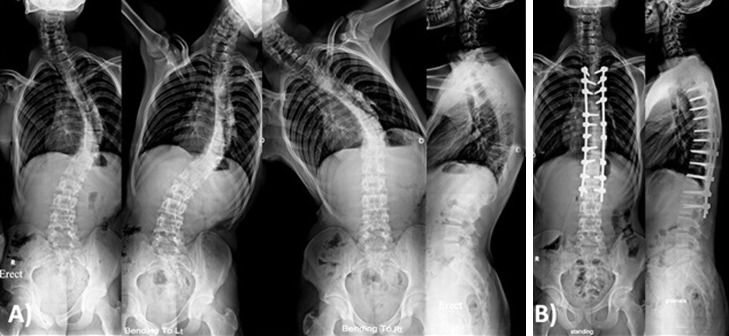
Male patient 17 years old with Lenke 1 a n. (A) Preoperative X-rays showing main thoracic curve of 50° that was corrected to 29° in side bending films with thoracic kyphosis of 40°. (B) One year postoperative X-rays showing posterior instrumentation from T4-L2 with main thoracic curve correction to 9° with thoracic kyphosis of 36°.
